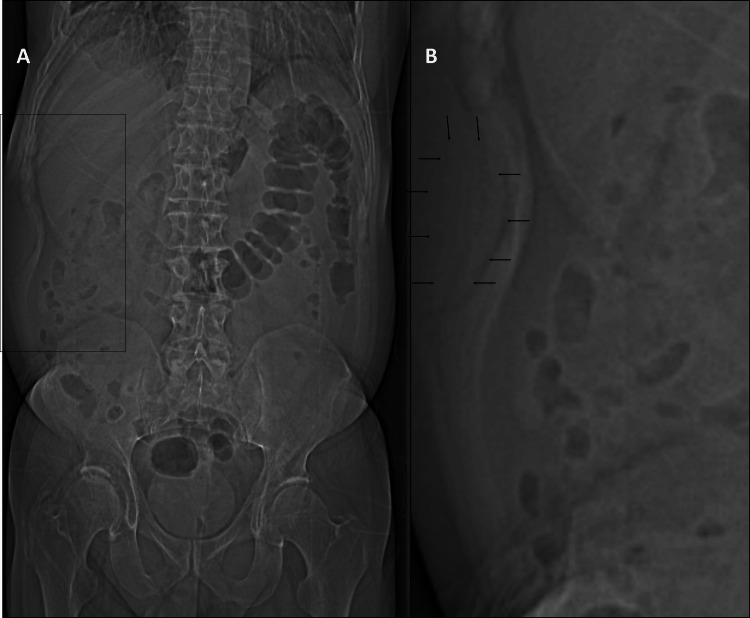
Scout image (abdominal radiograph from CT) of the abdominal wall mass. (A) The scout image demonstrated subtle soft tissue prominence along the right thoracoabdominal wall at the level of the right 12th rib, without evidence of underlying rib erosion or soft tissue calcification. (B) Magnified image highlighting the area of soft tissue prominence, suggestive of an underlying lesion (black arrows).
